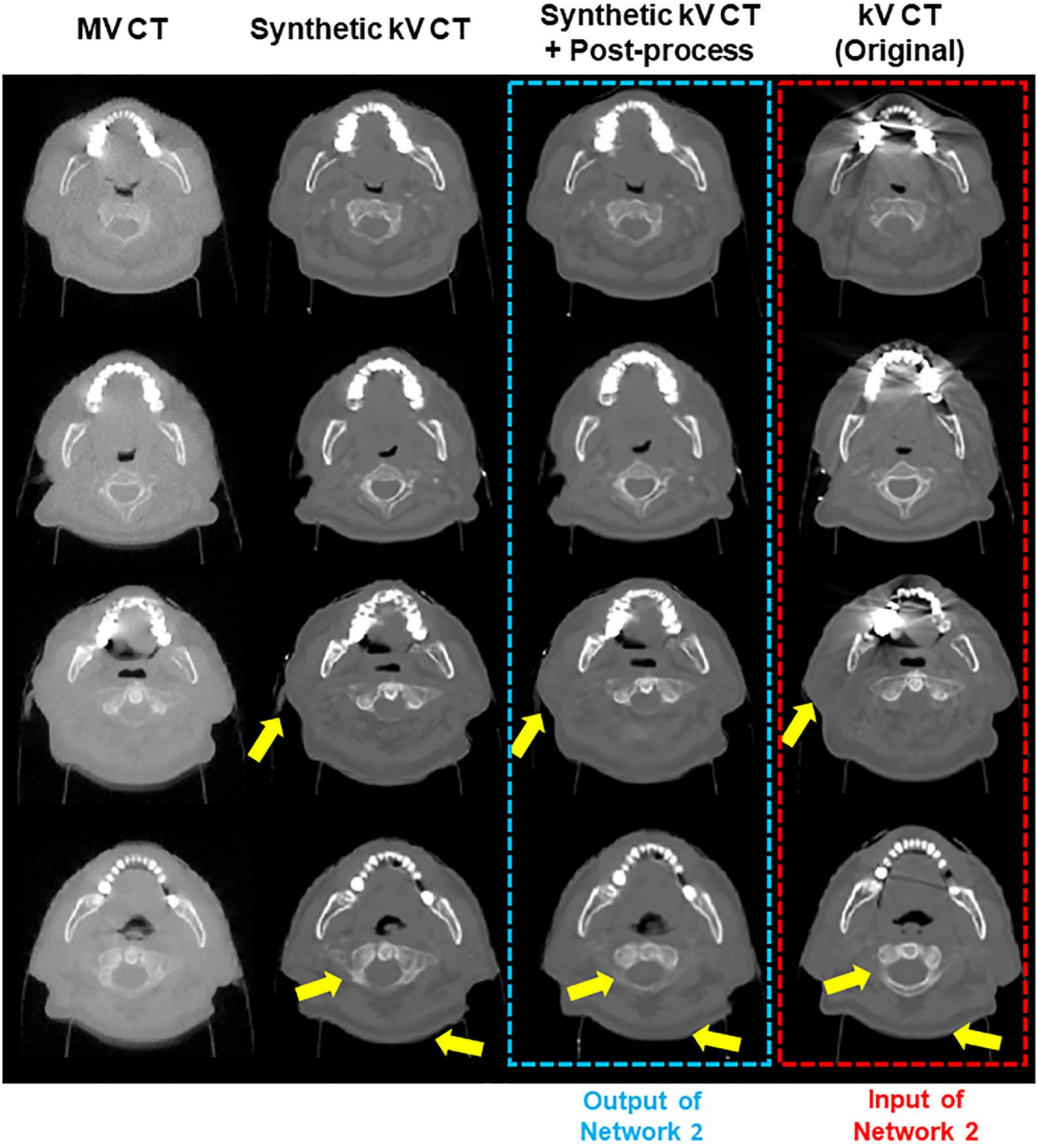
The results of Network1 (Synthetic kV CT) from MV CT and the images through additional processing (Synthetic kV CT + Additional Process) for 2D slices containing metal elements, compared against true metal-contaminated kV CT (MV CT [−1000 HU,750 HU], kV CT: [−1000 HU, 1500 HU]).
